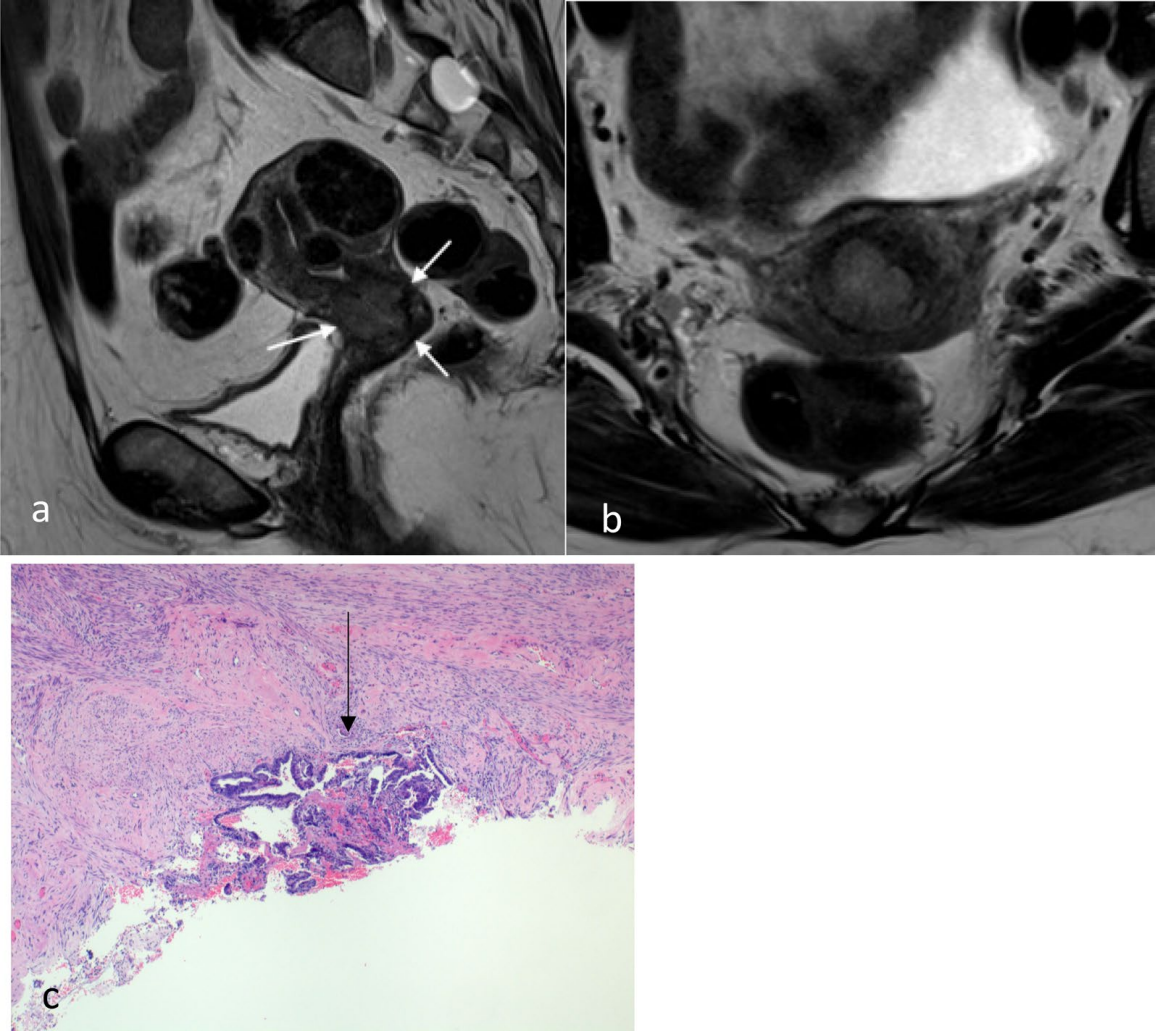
Stage II endometrial carcinoma with cervical stroma involvement. On sagittal (a) and axial (b) T2W MR imaging, there is intermediate T2 signal tumour (arrows) replacing the low signal dense cervical stromal tissue. In this case, the tumour involving the cervix is from a drop metastasis from a smaller primary in the endometrial cavity (not shown). Pathology (c) determines cervical invasion based on identification of the haphazardly arranged tumour cells (arrow) invading beyond the confines of cervical mucosa with surrounding stromal reaction
